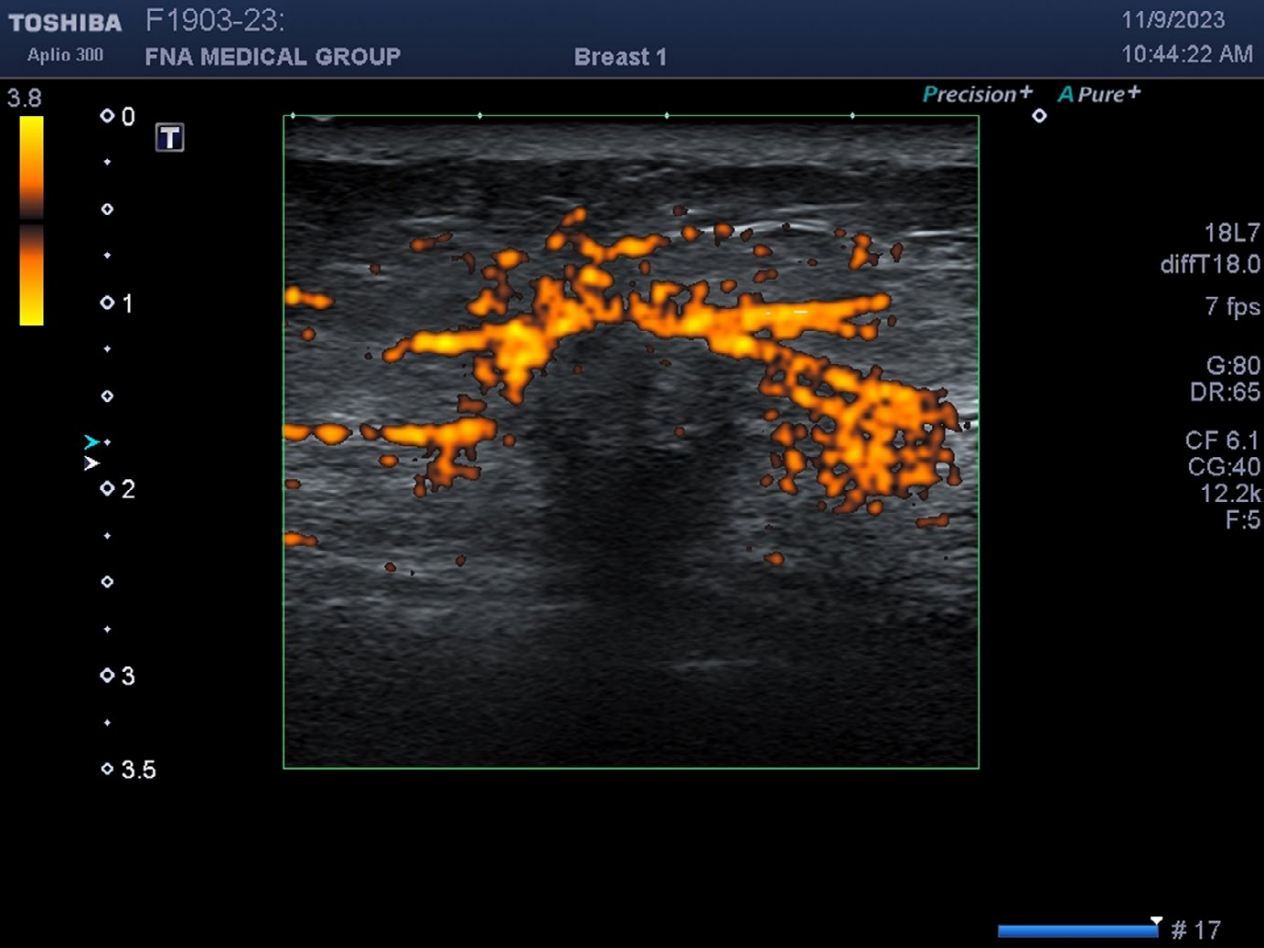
During power Doppler vocal fremitus, the patient says ‘eeeeee…’ loudly with power Doppler turned on. The tissue surrounding the mass vibrates and produces a Doppler signal. The mass does not vibrate and is outlined by an orange flash. This could help identify subtle breast masses.
